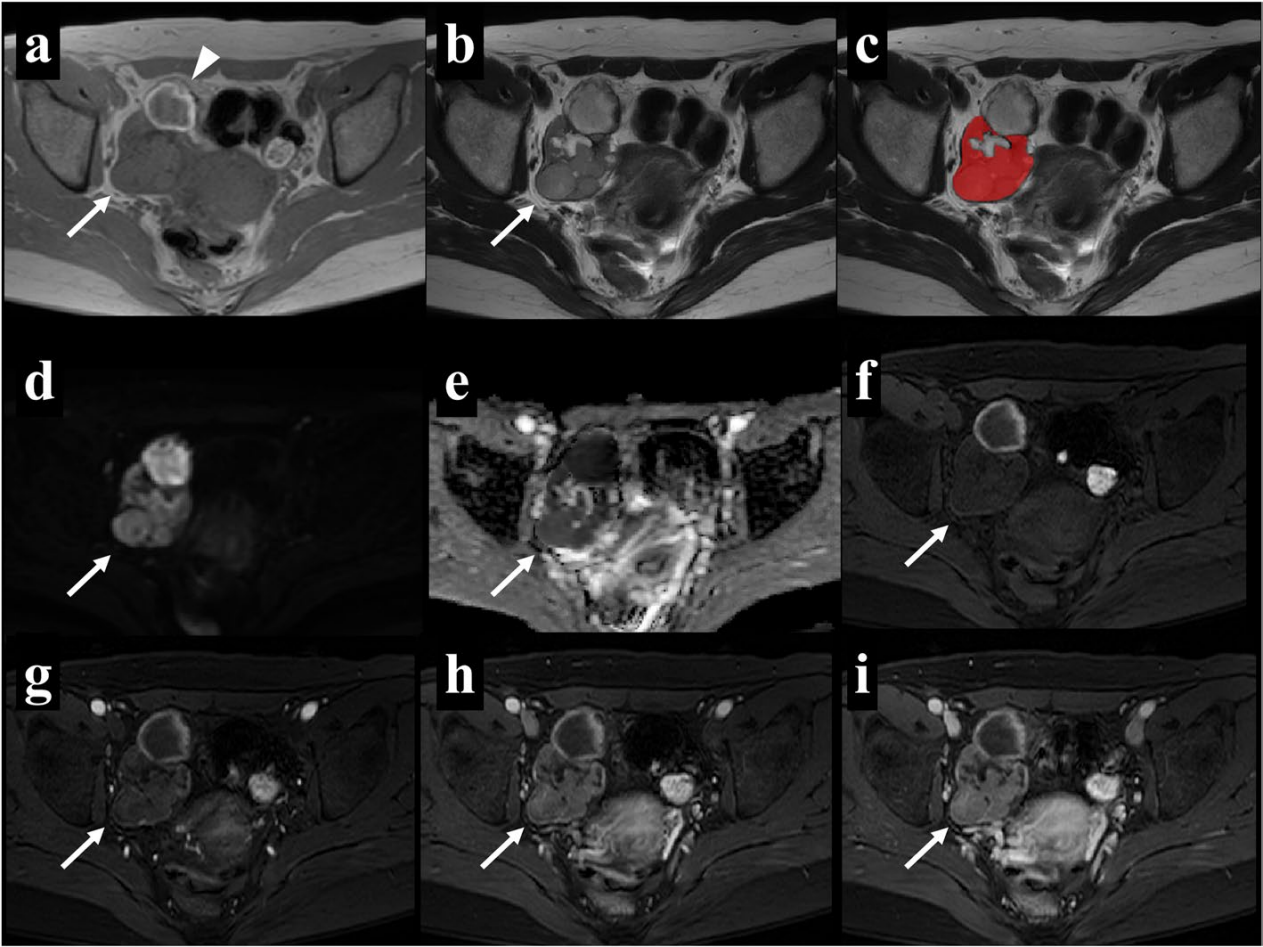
A 45-year-old woman with a right ovarian adult granulosa cell tumor. Axial T1WI (a) showed a hemorrhagic change in the dorsal part (arrowhead) and axial T2WI (b) showed a homogeneous isointense solid region with a partial cystic change. The region of interest (ROI) in the texture analysis (c) was delineated to contain the outline borders of the solid region of the tumor, and the cystic region was avoided as much as possible. Axial DWI (d) showed a hyperintense solid area, and the apparent diffusion coefficient (ADC) map (e) showed that the average ADC value of this area was 0.84 × 10^−3^ mm^2^/s. In multiphasic contrast-enhanced fat-suppressed T1-weighted MRI (f-i), the mass mainly showed mild enhancement in all contrast phases
